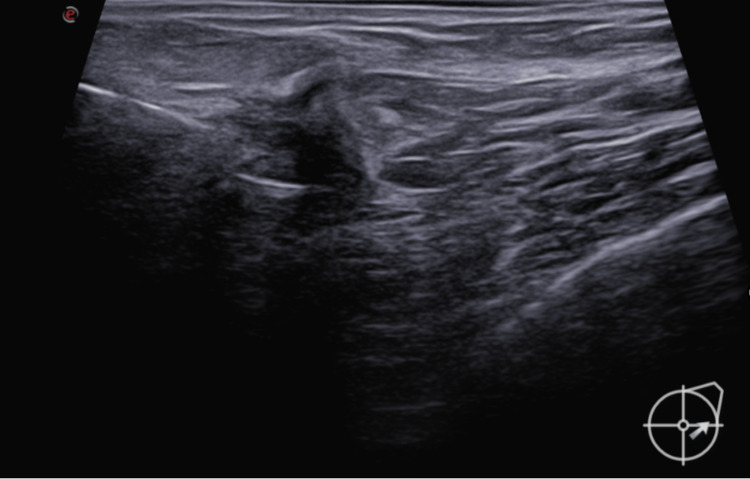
RFID usage Under local anaesthesia, the introducer needle with the notch facing upwards ~(like a step defect) is introduced within the tumour under ultrasound guidance. RFID: radio-frequency identifier device
